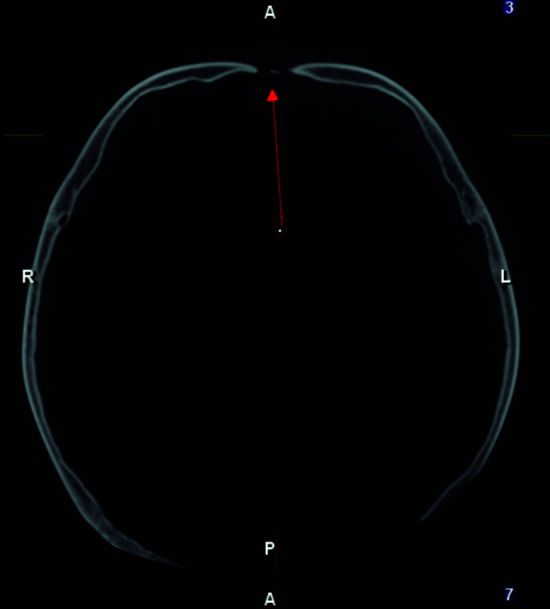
Axial CBCT section of a patient with CCD showing the open frontal suture.
